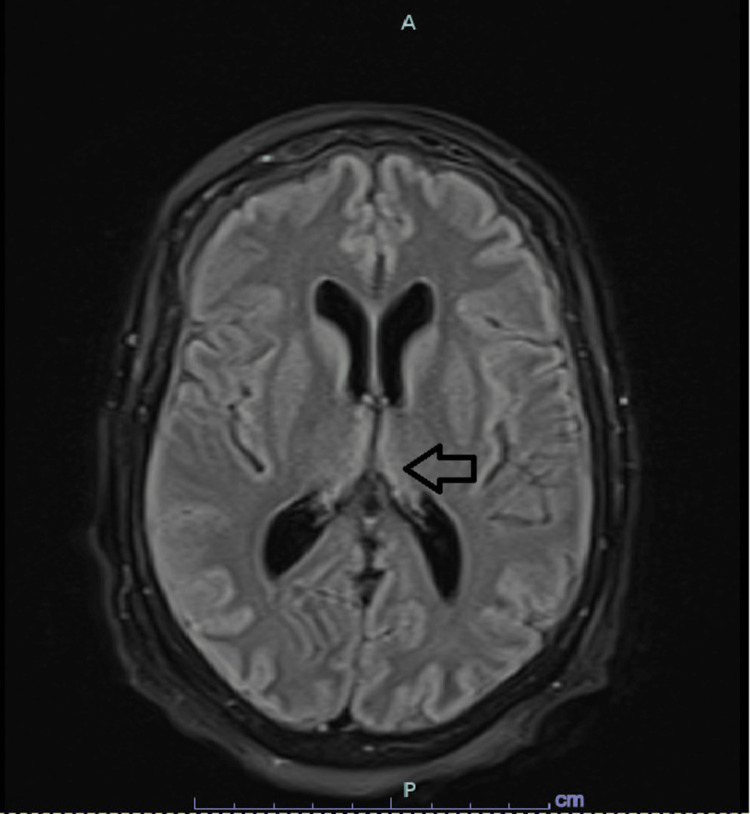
Follow-up MRI MRI of the brain obtained after initial thiamine replacement showing relative improvement in bilateral thalami hyperintensities (arrow).
